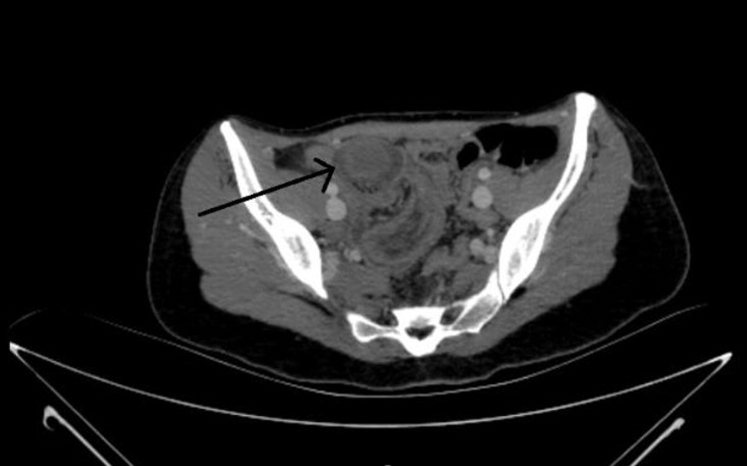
Axial view of the 3-layered structure in the ileocecal region suggesting intussusception on the patient's computed tomography (ring structure at the tip of the black arrow).
